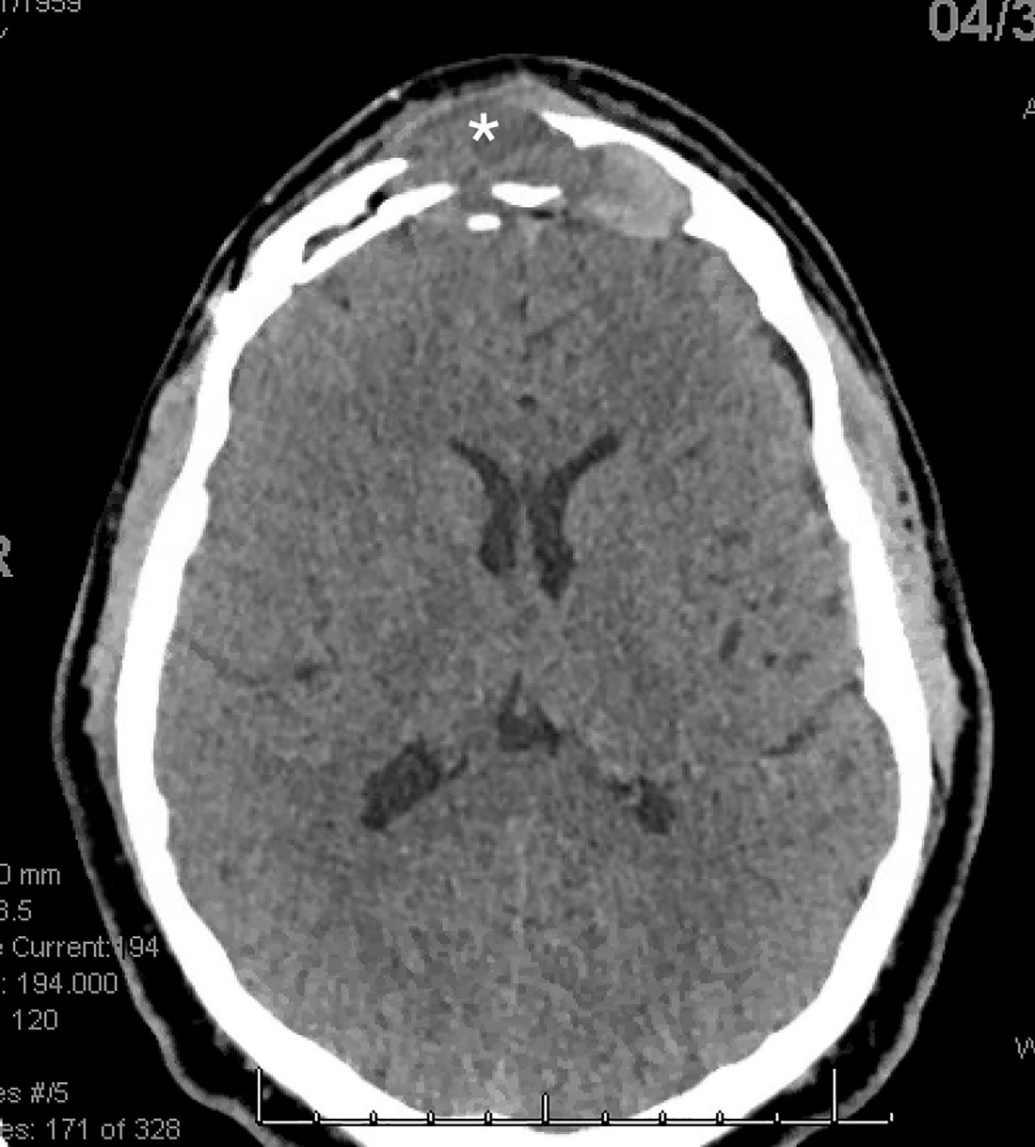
Computed tomography imaging demonstrating a soft tissue mass (*) extending through the frontal sinus and the right frontal lobe.
